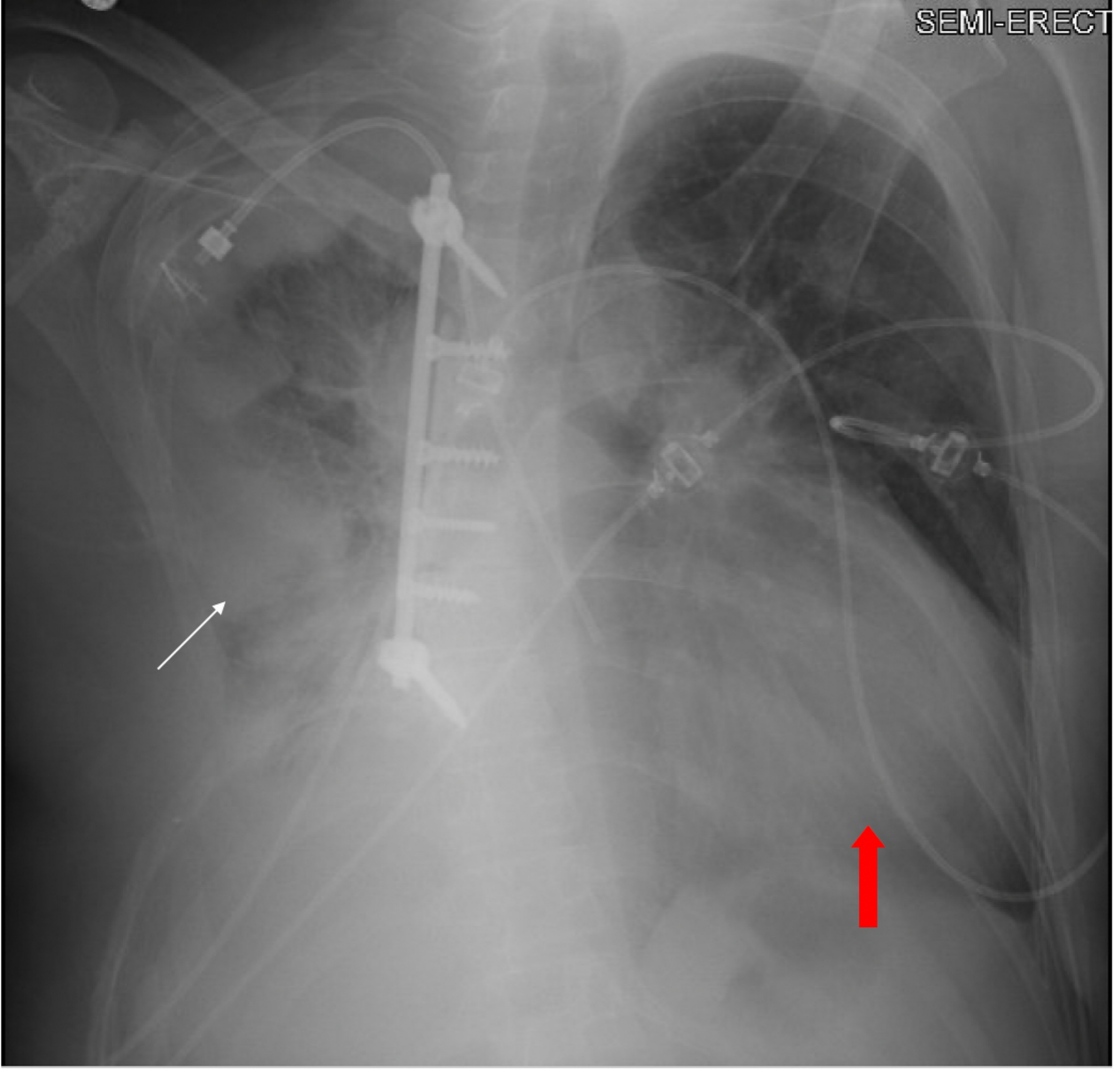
Chest X-ray showing enlarged cardiac silhouette (red arrow) as well as right hemithorax status post chest wall resection with remnant neoplastic process (white arrow)
